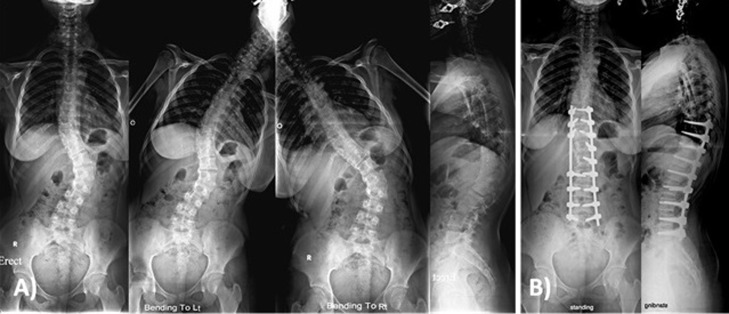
Female patient 16 years old with Lenke 5 c +. (A) Preoperative X-rays showing lumbar curve of 56° that was corrected to 41° and main thoracic curve of 28° that was corrected to 20° in side bending films, with thoracic kyphosis of 48°. (B) Two years postoperative X-rays showing posterior correction and fusion of the lumbar curve only from T9-L5 with lumbar curve correction to 10° and spontaneous main thoracic curve correction to 14° with thoracic kyphosis of 38°.
